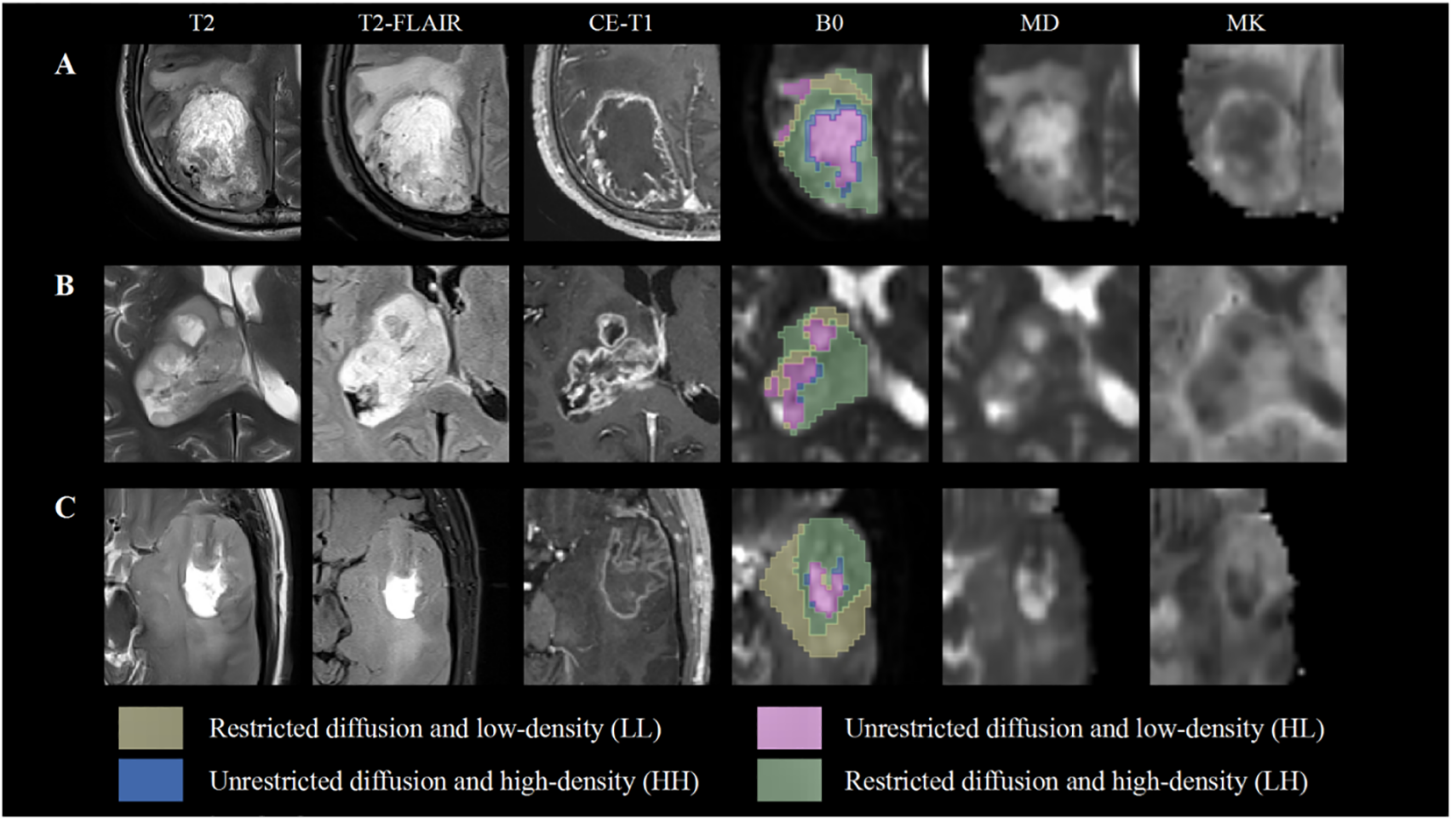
Subhabitats of three participants on different images. The B0 map shows four spatial habitats. The LL and HL habitats have a high level of overlap with peritumoral edema and cystic components compared with conventional MRI, mean diffusivity (MD) and mean kurtosis (MK) maps. (A, B) Show World Health Organization (WHO) grade IV glioblastoma with IDH wild type. (C) Indicates a WHO grade III oligodendroglioma with IDH mutation.
